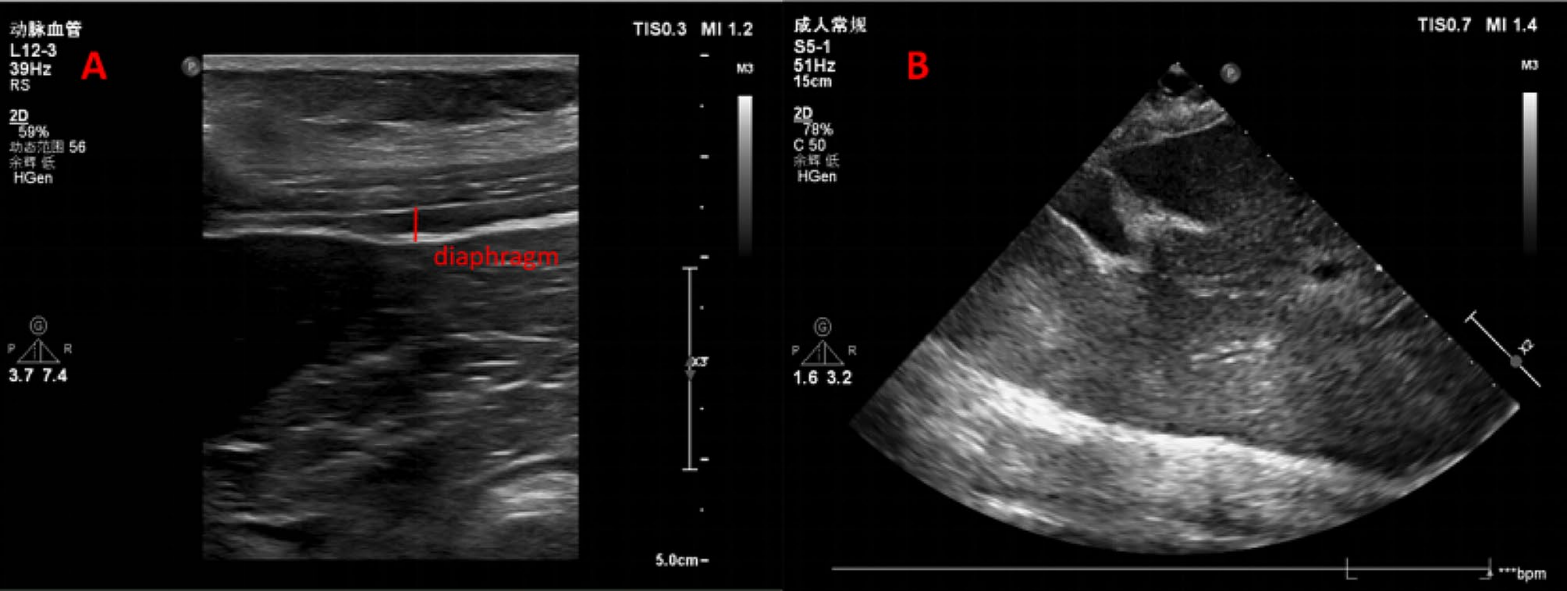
Diaphragm ultrasound images. A DTF measured by high-frequency probe in horizontal position; B DE measured by low-frequency probe in semi-recumbent position
